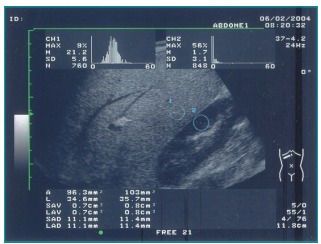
Ultrasonography of the liver in a patient with hepatic steatosis. The
